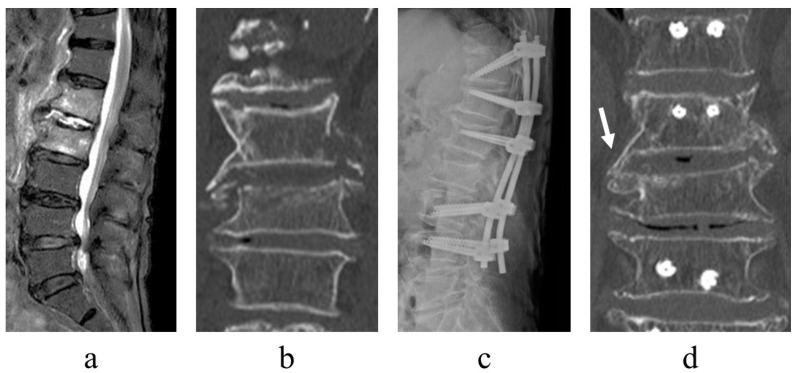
Image of a representative case of postoperative lateral bony bridge. An 87-year-old woman, L1/2 pyogenic spondylitis. Short tau inversion recovery on magnetic resonance imaging revealed signal changes in the vertebral body (a), and computed tomography (CT) demonstrated bone destruction and resorption of the L2 vertebral cranial endplate ((b), coronal view). The patient experienced persistent back pain and underwent posterior fixation of T11–L4 with percutaneous pedicle screw ((c), plain radiograph of thoracolumbar lateral view). One year postoperatively, a lateral bony bridge was obtained between the lateral L1 and L2 vertebrae ((d), CT coronal vie9w, arrow).
